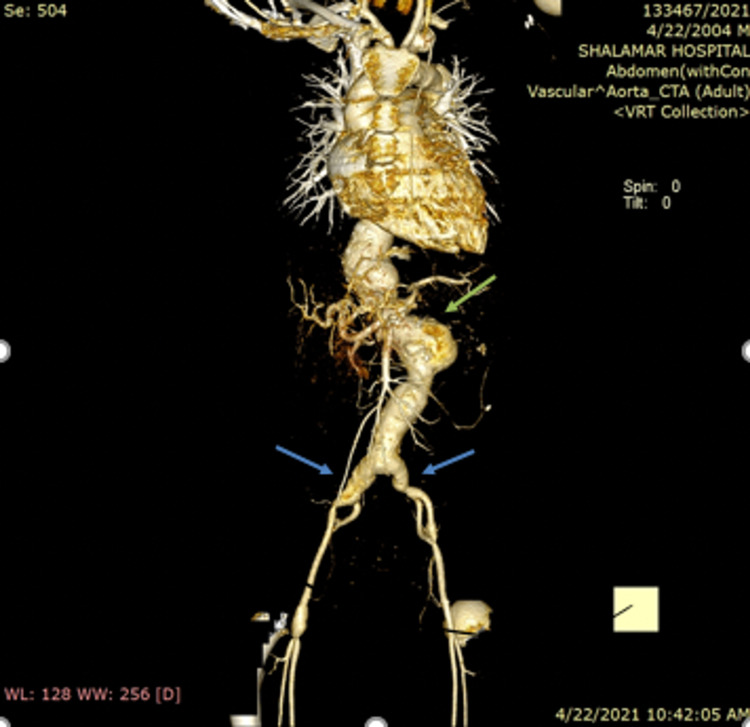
3D constructed view of CT angiogram showing thoracoabdominal aortic aneurysm involving both common iliac veins (blue arrows) and eccentric saccular outpouching of the pararenal part (green arrows)
